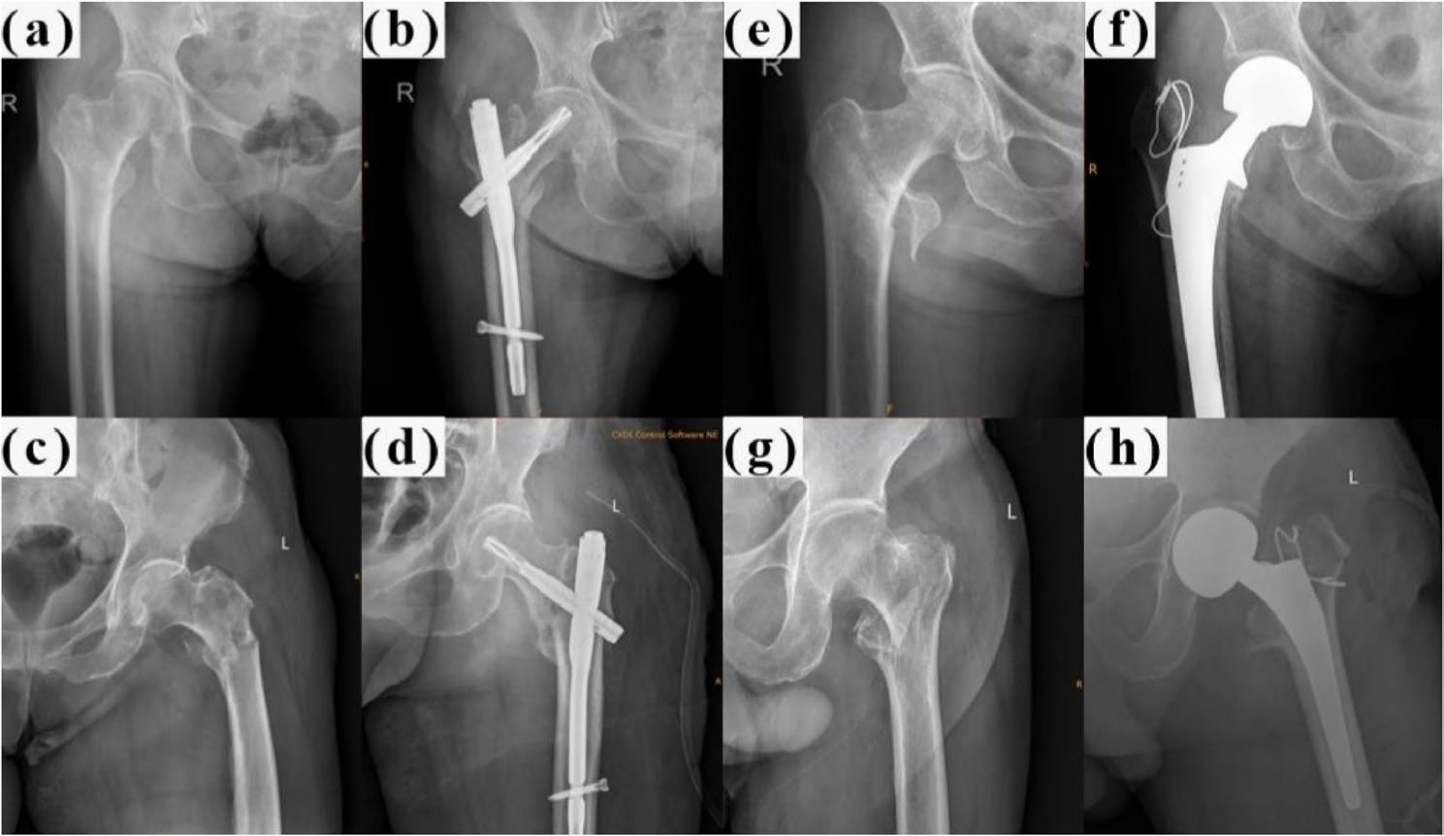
Preoperative and postoperative radiographs of various AO/OTA classifications of ITF treated by PFNA and HA. a Preoperative radiograph of a stable ITF (A1.1). b Postoperative radiograph of a stable ITF (A1.1) treated with PFNA. c Preoperative radiograph of an unstable ITF (A3). d Postoperative radiograph of an unstable ITF (A3) treated with PFNA. e Preoperative radiograph of a stable ITF (A1.3). f Postoperative radiograph of a stable ITF (A1.3) treated with HA. g Preoperative radiograph of an unstable ITF (A2.3). h Postoperative radiograph of an unstable ITF (A2.3) treated with HA. AO/OTA: AO Foundation/Orthopedic Trauma Association classification system; ITF: intertrochanteric fracture; PFNA: proximal femoral nail antirotation; HA: hemiarthroplasty
